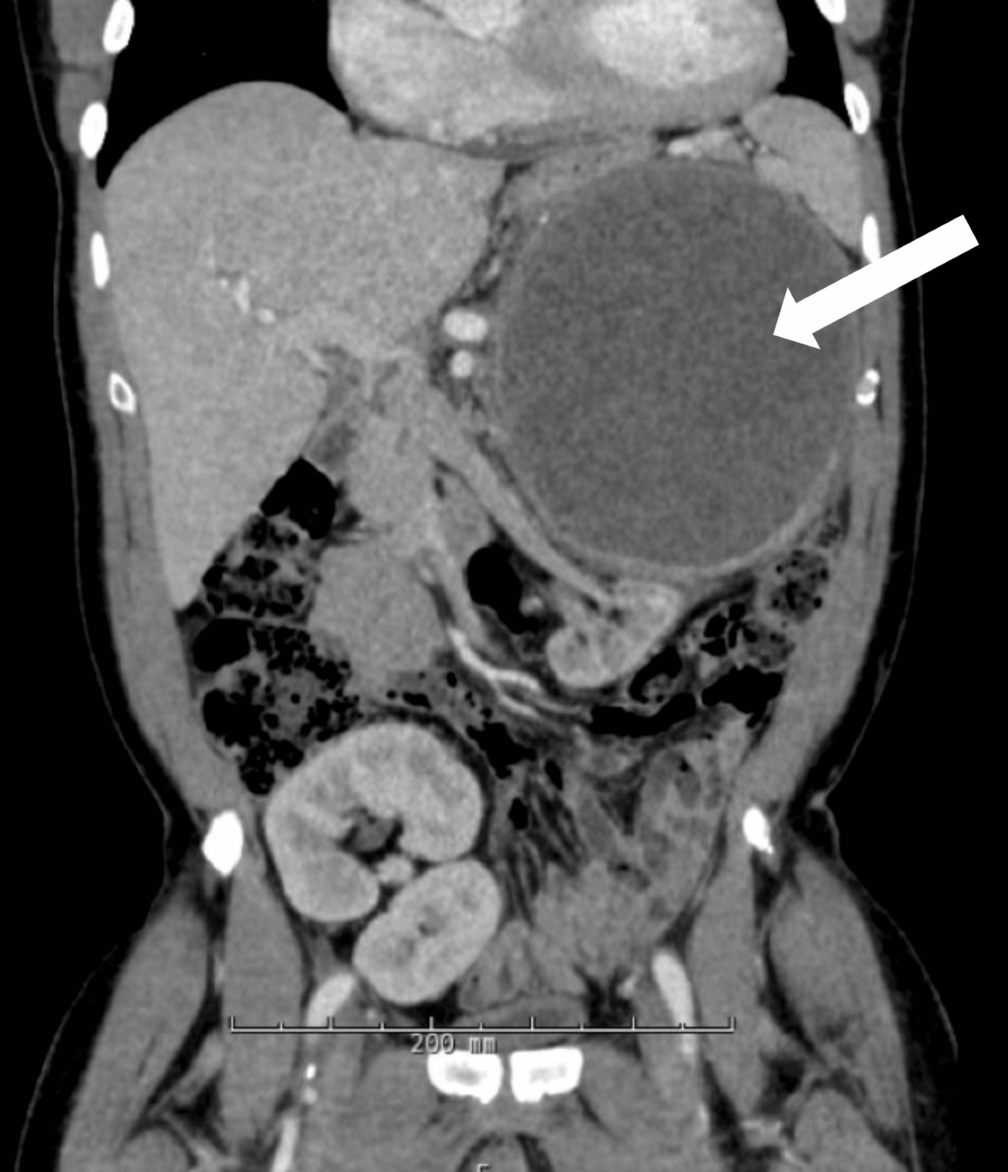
CT scan demonstrating displacement of the left native kidney with its vasculature, as well as compression of the pancreas, spleen, and stomach. White arrow indicates a cystic lesion with internal heterogeneity and mass effect, meeting the criteria for a complex cyst, causing displacement of the left native kidney, pancreas, spleen, and stomach
